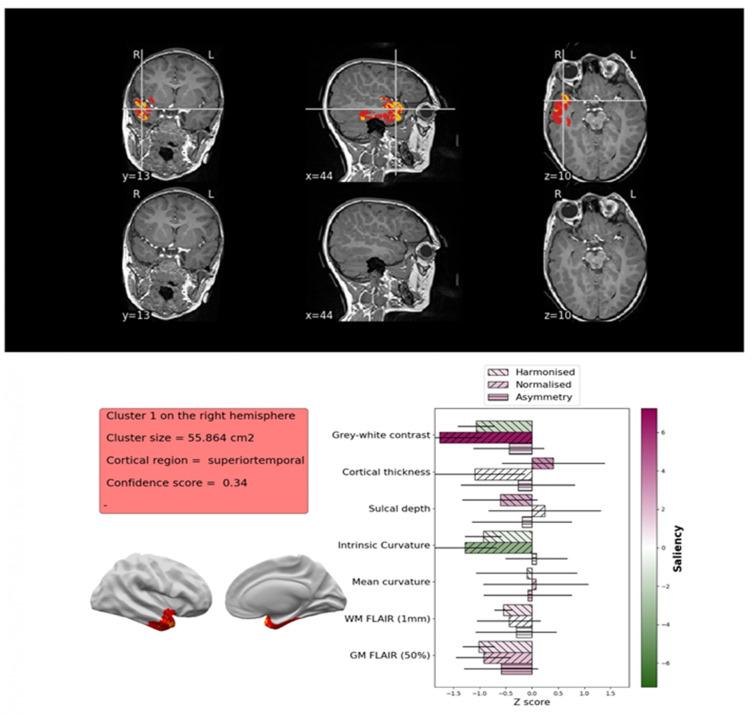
Patient’s MRI exam was processed through the MELD surface-based FCD detection algorithm, and a large FCD cluster was detected in the right temporal lobe. Particularly, the 3D T1 MPRAGE and FLAIR sequences were processed using FreeSurfer [120] to extract the following features: grey-white matter intensity contrast, cortical thickness, sulcal depth, intrinsic curvature, mean curvature, and FLAIR intensity sampled at different intracortical and subcortical depths. To ensure robustness, these features underwent several pre-processing steps. Specifically, harmonization was performed to correct for site- and scanner-related differences; normalization was performed to account for intra- and inter-subject variability; and asymmetry analysis was performed to enhance detection of inter-hemispheric differences. Prior to application on the patient, the MELD Graph U-Net model was trained on a large multi-centre dataset and benchmarked against an existing algorithm [121]. to enable precise identification of the lesion location, size, characteristics, and feature saliency, namely the relative importance of the MRI features. (https://github.com/MELDProject (accessed on 20 July 2025)) *MELD (Multi-centre Epilepsy Lesion Detection); FCD (Focal Cortical Dysplasia); MPRAGE (Magnetisation Prepared Rapid Gradient Echo Imaging), FLAIR (fluid-attenuated inversion recovery)*.
